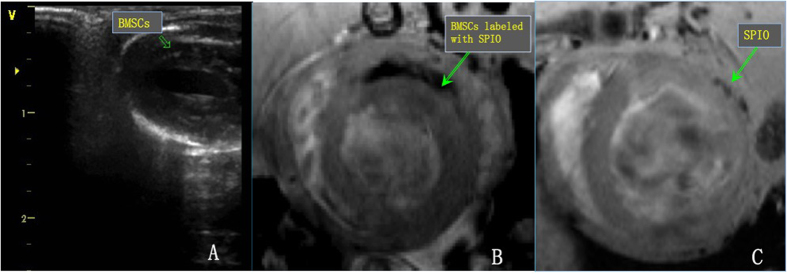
Bone mesenchymal stem cell (BMSC) tracking *in vivo*. (A) On transthoracic echocardiography, BMSCs at the border of the infarcted myocardium appeared as hyper-echoic lesions. On the cardiac MR imaging, SPIO-labelled BMSCs were visible as a hypo-intense area with sharp borders at the injection site after transplantation (B), but the SPIO signals became nearly undetectable after 14 days (C).
